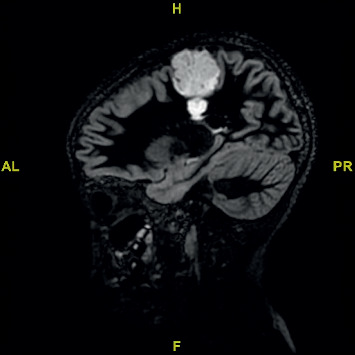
Sagittal flair MRI demonstrating tumour in the primary motor cortex.
